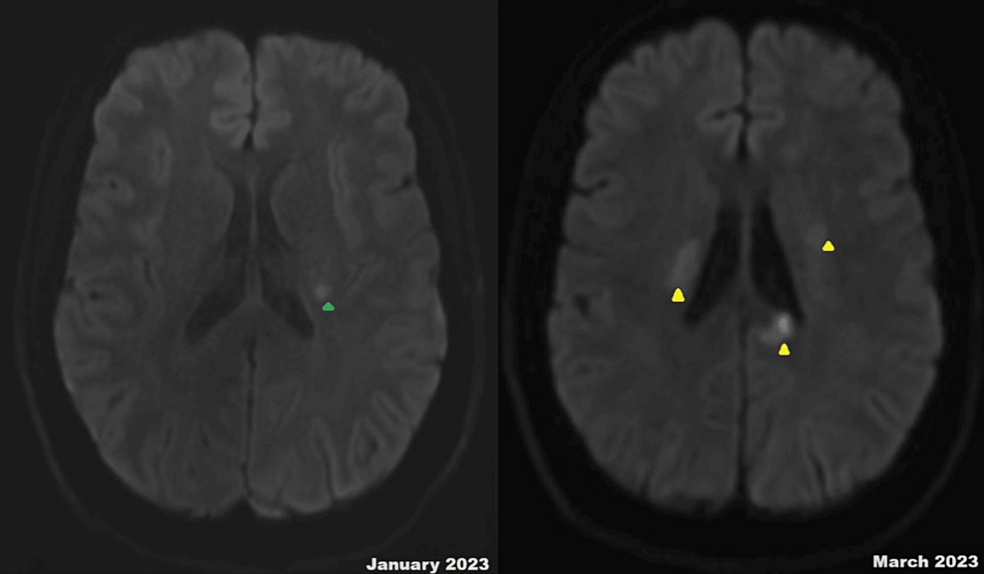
MRI of the head demonstrating the increasing cerebral infarct burden over the course of two months. DWI sequence of the MRI of the head (January 2023) shows a small cerebral infarct in the left corona radiata (green arrow) and left midbrain (not shown). DWI sequence of the MRI of the head (March 2023) shows numerous foci of cerebral infarction (right and left corona radiata, as shown with yellow arrow) as well as bilateral frontal, left temporal, and parietal cortices (not shown). DWI: diffusion-weighted imaging; MRI: magnetic resonance imaging.
